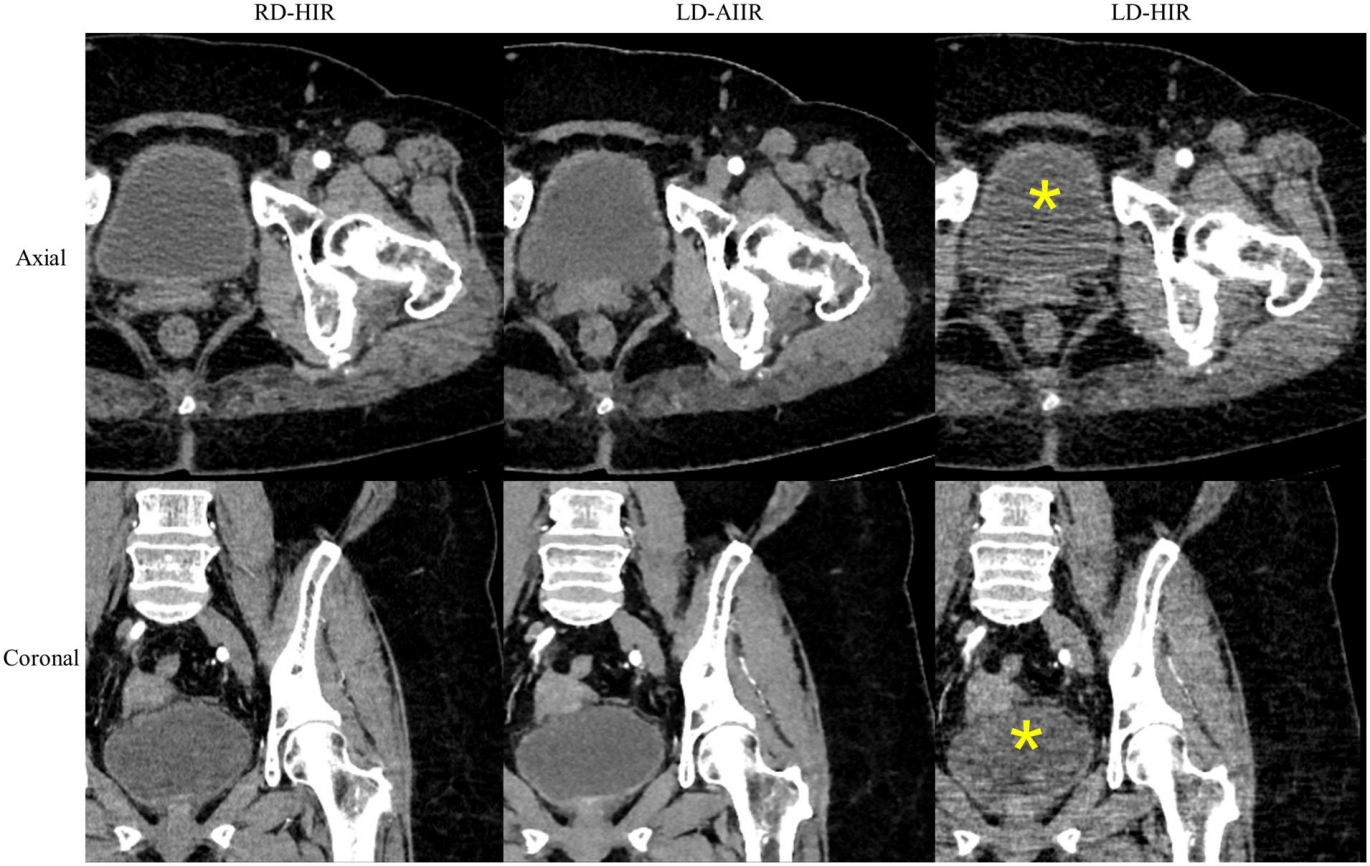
Corticomedullary phase CT images of 63-year-old woman at routine-dose with hybrid iterative reconstruction (RD-HIR), low-dose with artificial intelligence iterative reconstruction (LD-AIIR) and with hybrid iterative reconstruction (LD-HIR), where LD-HIR images failed to meet clinical requirements due to severe streak artefacts around the bladder (yellow *).
